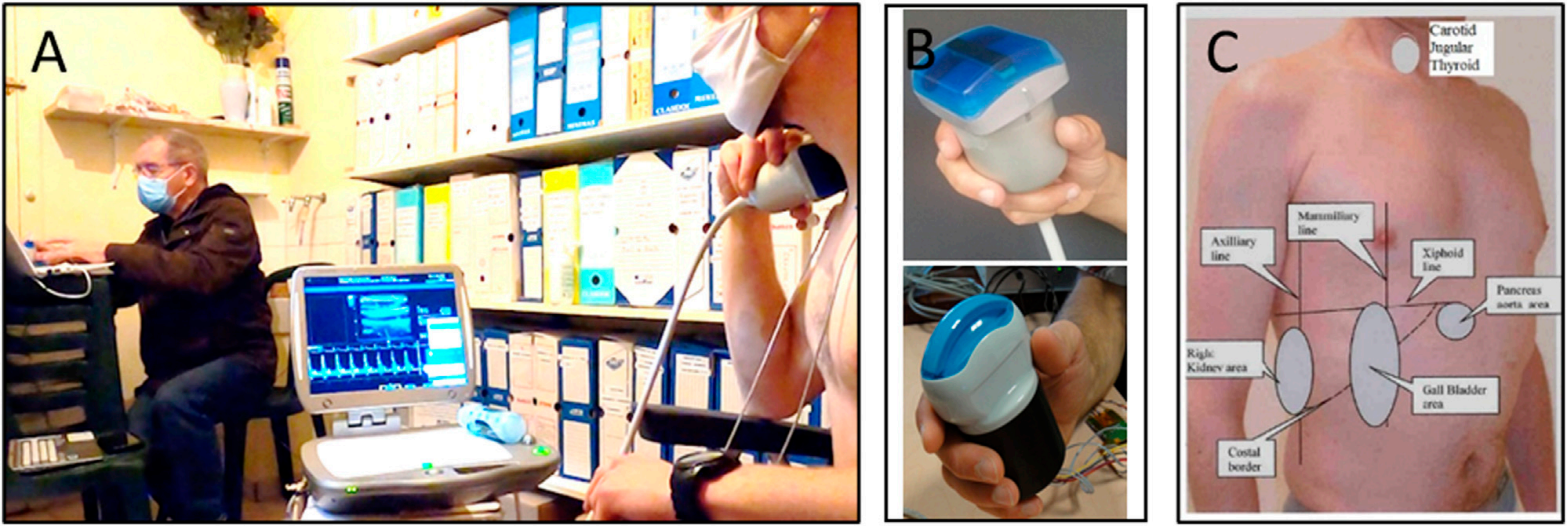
Images show the experimental setup for the ultrasound data collection. In panel (A) the participant is holding the motorized probe in place (right) while the expert sonographer (left) teleoperates the system to acquire images for analysis. Panel (B) depicts the 3.5 MHz and 17 MHz motorized probes used for the investigation. Finally, panel (C) shows the map of the various acoustic windows of each organ (ex., gall bladder, kidney) and landmarks (ex., mamillary, axillary line) used for the ultrasound assessments that was provided to the participant as reference for proper probe placement.
